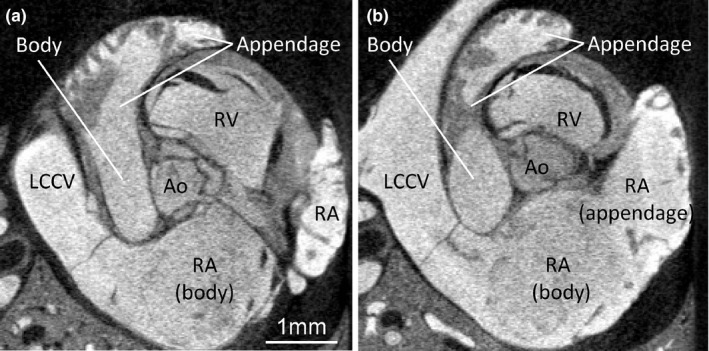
Left atrium. (a) Image from micro‐CT showing the left atrium has a smooth‐walled body and a proportionally large trabeculated appendage. (b) Trabeculations of the left atrium are more coarse than those of the right atrium (RA). Ao, aorta; LCCV, left cranial caval vein; RV, right ventricle. Both images are approximately in the transverse plane
